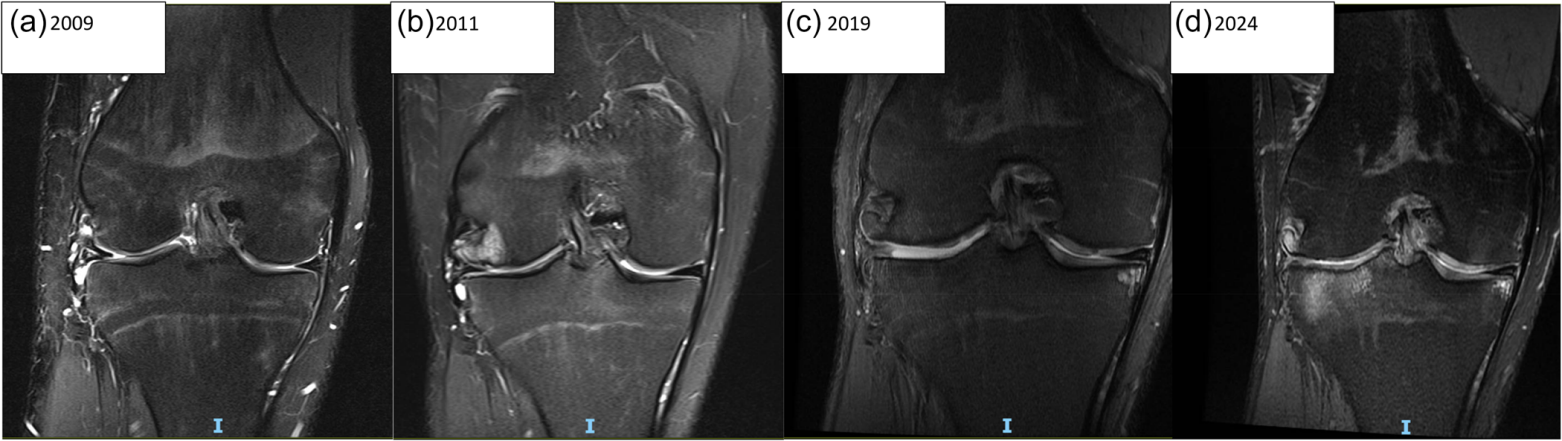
MRI Sequence of right knee injury in a professional handball player. (a) Lateral meniscal injury followed by meniscal repair. (b) Subchondral bone collapse after treatment. (c) Tricompartmental osteoarthritis. (d) Evolution of tricompartmental osteoarthritis 15 years later.
